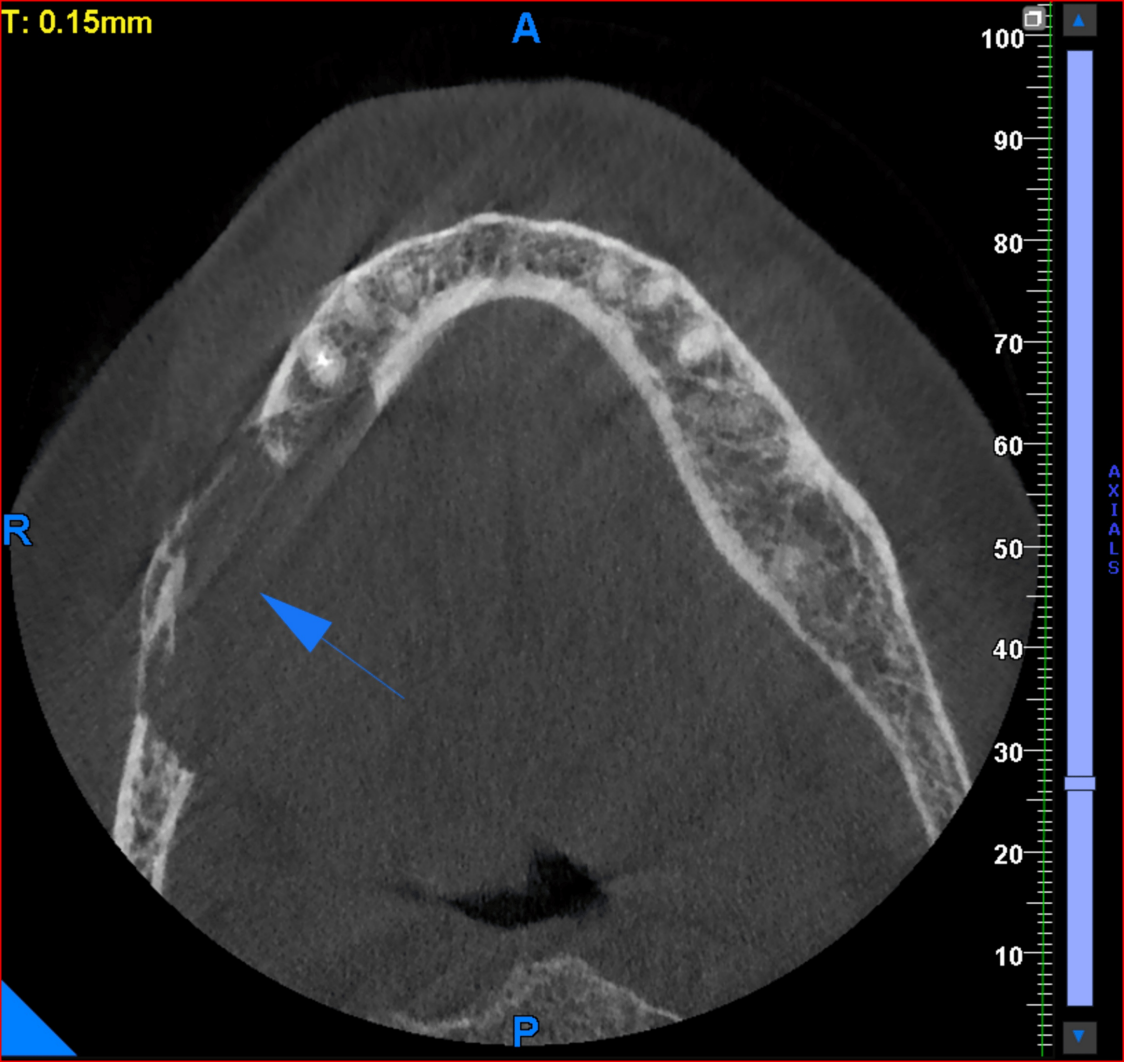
CBCT: axial section The arrow indicates an expansile osteolytic lesion with irregular margins involving the alveolar process of the right mandible, with erosion of both buccal and lingual cortical plates. CBCT, cone-beam computed tomography
